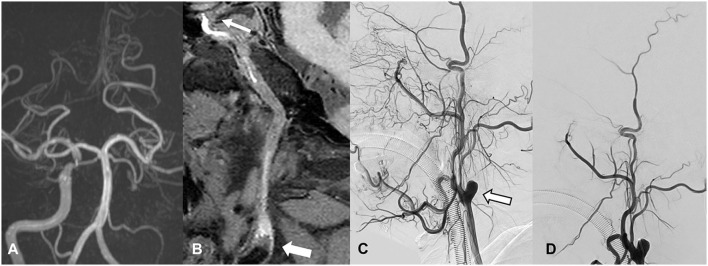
A 49-year-old man with progressive visual loss of left eye. (A) Long segmental occlusion of left internal carotid artery (ICA) is detected by TOF-MRA. (B) HR-MRI shows occlusion with blunt stump and involving ophthalmic artery segment and above. This patient had scored as point 2 before recanalization. (C) Lateral images of DSA confirm the blunt stump and no reversed flow of distal ICA. (D) The recanalization attempt is failed because catheter cannot pass the stump.
